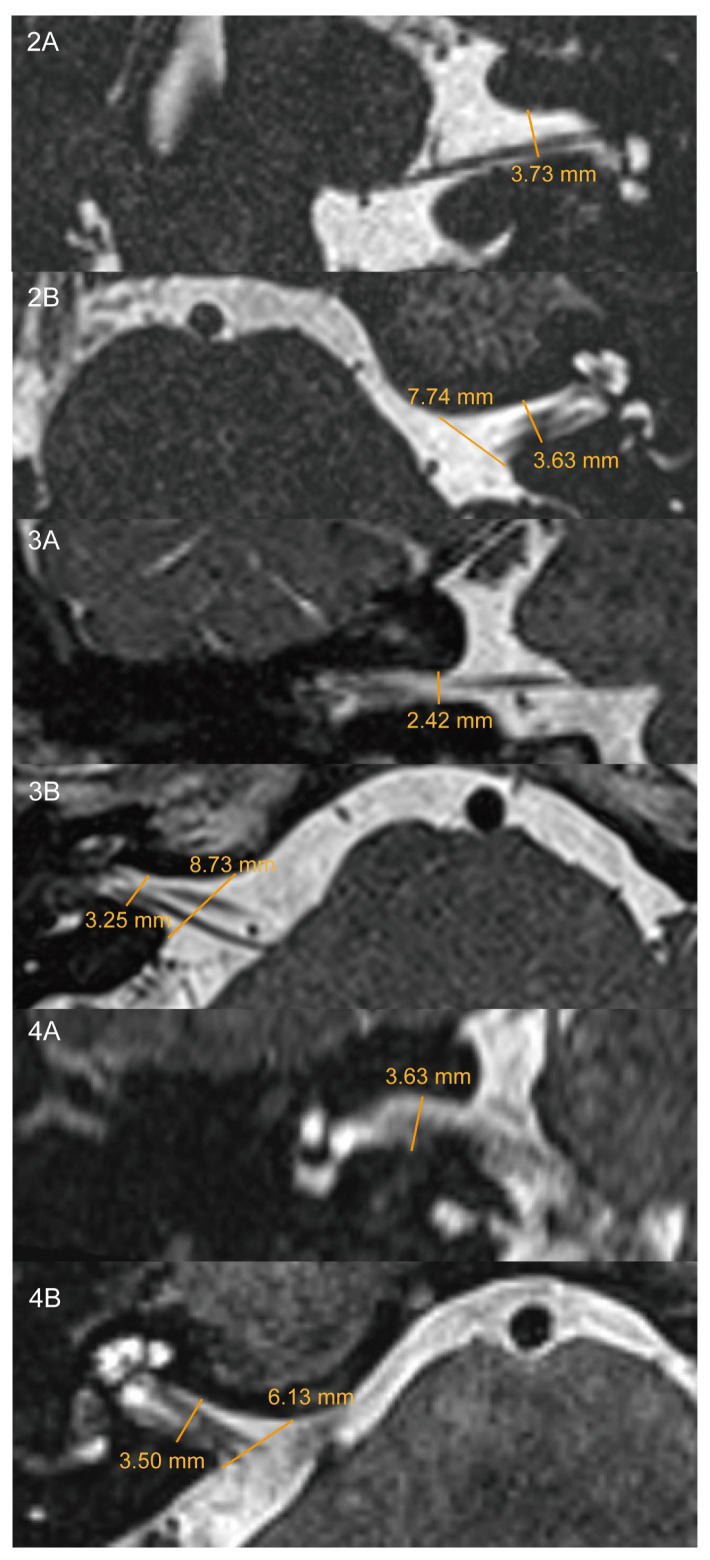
Coronal and axial high-resolution T2-weighted MRI images showing IAC measurements in Cases 2–4. Coronal images (2A,3A,4A) demonstrate IAC widths of 3.73 mm, 2.42 mm, and 3.63 mm, respectively. Axial images (2B,3B,4B) show corresponding axis widths of 3.63 mm, 3.25 mm, and 3.50 mm, respectively. IAC, internal auditory canal.
